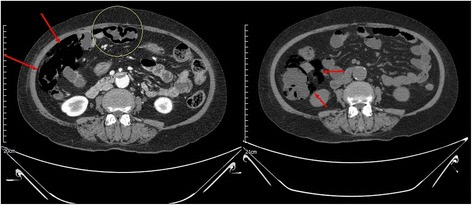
Abdominal CT at initial presentation. Note the air collection within ileal loops and colonic wall on initial CT (Left). PCI was severe, thus air-containing cysts (arrows and circle) were distributed at both of mesenteric and anti-mesenteric border. On the follow-up CT (Right) taken 1 week later, improving PCI was observed. Cysts at mesenteric border and near mesenteric vessels were predominant (arrows)
